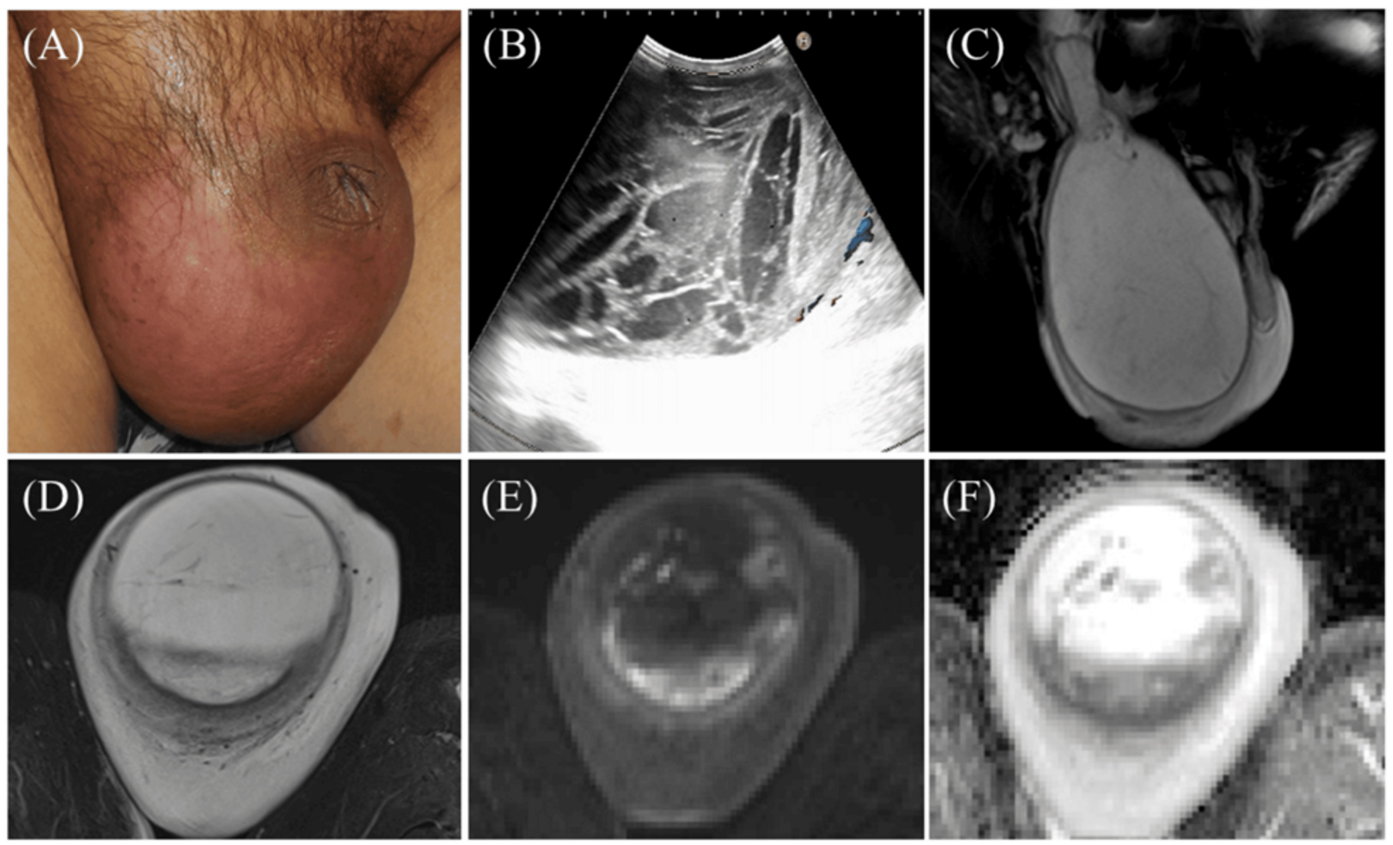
Imaging of the scrotum (A) The scrotum, erythematous predominantly on the right side, is markedly swollen to approximately the size of a clenched fist. (B) Scrotal ultrasound revealing multiple septa within the scrotum, with no well-defined abscess cavity suitable for drainage. (C) Coronal fat-suppressed T2-weighted image showing a large cystic lesion (size, 22 cm) extending from the right inguinal canal into the scrotum. (D) Axial fat-suppressed T2-weighted image showing a bilocular lesion, with a thickened cyst wall and internal low-signal linear structures suggestive of septations. Surrounding the cyst wall, edematous changes in the adjacent soft tissue are also observed. (E) Diffusion-weighted imaging (b = 1000 s/mm^2^) demonstrating increased signal intensity predominantly along the cyst wall, appearing as punctate and sheet-like areas. (F) The corresponding apparent diffusion coefficient map shows low signal intensity in these regions, indicating restricted diffusion consistent with an abscess.
